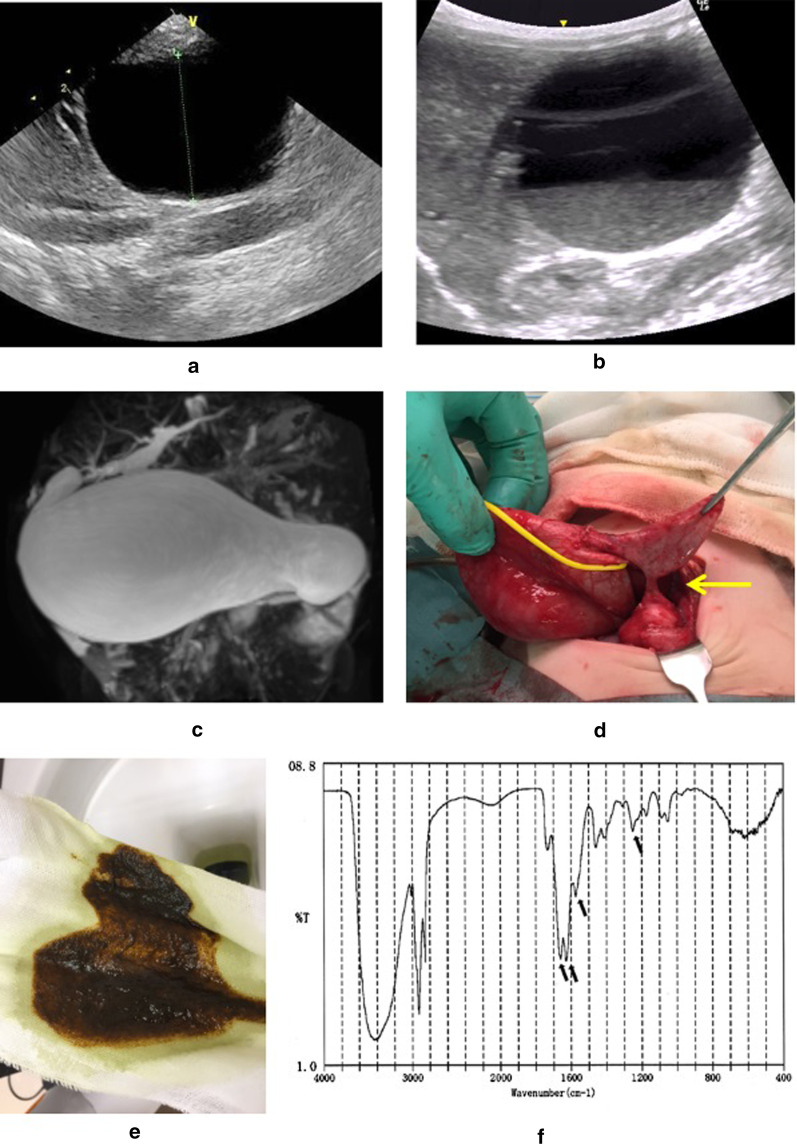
Data of Case 1. a Ultrasonography on day 2 showed a large choledochal cyst but no debris. b Ultrasonography on day 45 revealed debris in the cyst. c, d Magnetic resonance imaging and surgical findings confirmed a huge cyst and a narrow segment (arrow). e, f Infrared absorption spectra of the debris. Four major absorption bands (arrows) were characteristic of calcium bilirubinate
